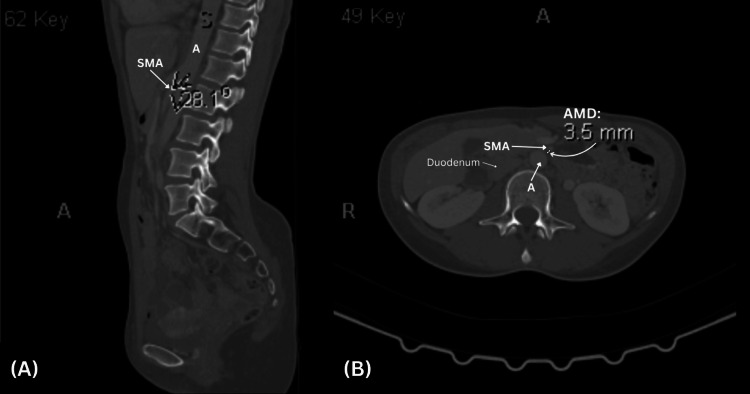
CT abdomen and pelvis with contrast revealing narrowing of the duodenum between the aorta and SMA. (A) Contrast-enhanced CT in sagittal projection demonstrates an aortomesenteric angle of 28° between the superior mesenteric artery (SMA) and the aorta (labeled ‘A’). (B) Contrast-enhanced CT in axial projection demonstrates an aortomesenteric distance (AMD) of 3.5 mm between the SMA and the aorta.
